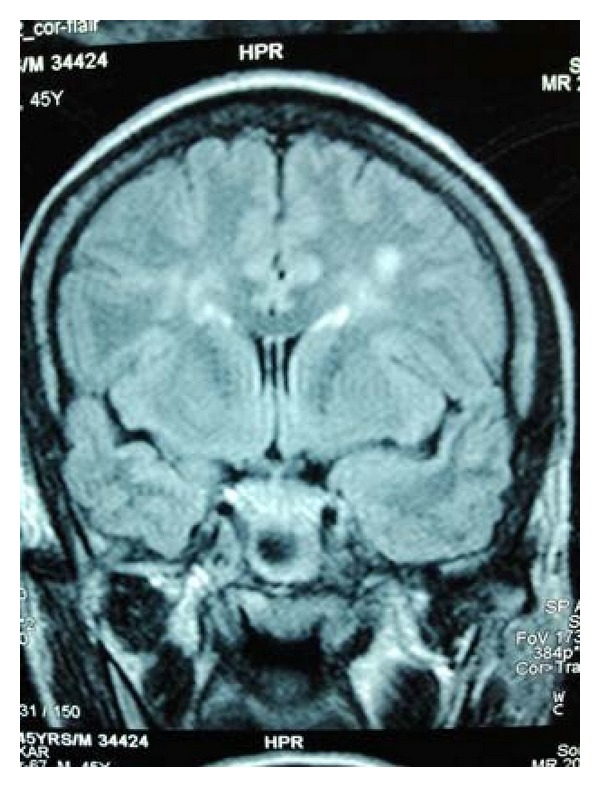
Magnetic resonance image of the brain showing nonspecific white matter changes.
